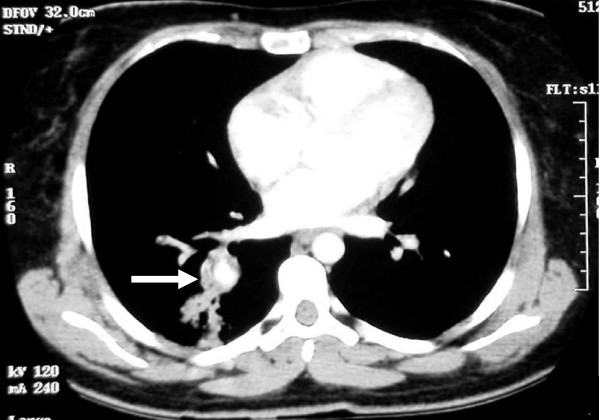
Contrast-enhanced computed tomography scans of the chest demonstrated a right lower lobe pulmonary artery aneurysm (white arrow).
